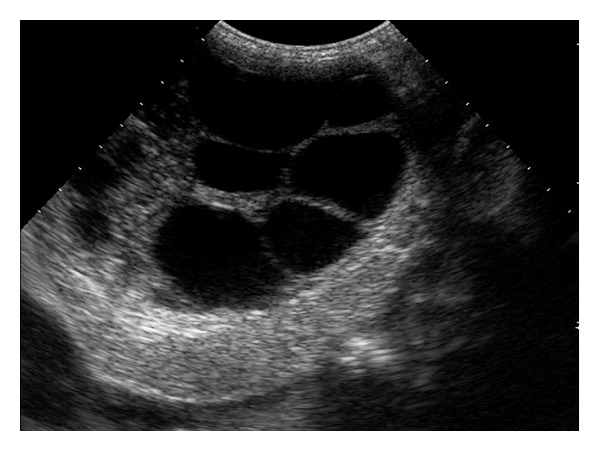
Ultrasonography scan of liver showing multilocular anechoic cystic lesion made up of multiple cysts that ranged from 10 to 45 mm in diameter occupying whole left hepatic lobe. Biliary and vascular structures are displaced but not involved.
